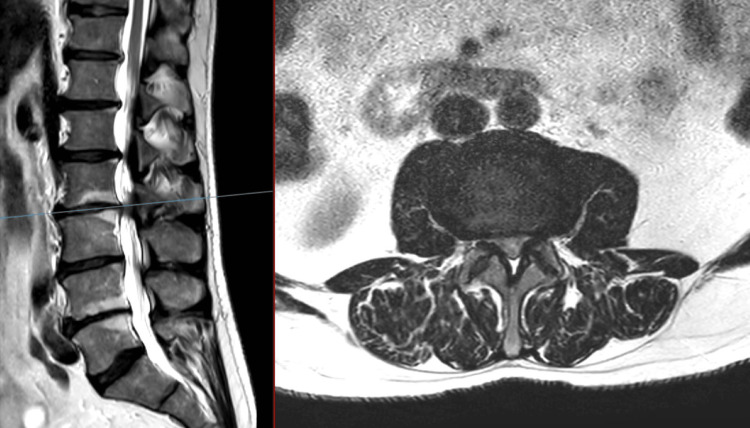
T2 MRI of the lumbar spine: sagittal (left) and axial (right) at L2/3 Moderate central spinal stenosis was noted, along with severe lateral recess stenosis. Effacement of the CSF and compression of the cauda equina were seen. Bilateral facet hypertrophy (right > left) was noted. CSF: cerebrospinal fluid
